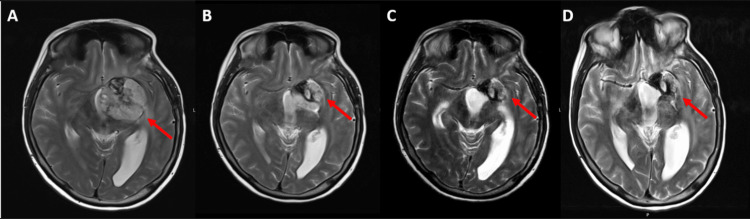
Sequential brain MRI The lesion-reducing effect of dabrafenib plus trametinib (arrows) A - Before the beginning of treatment; B - Three months after treatment; C - Six months after treatment; D - Nine months after treatment
